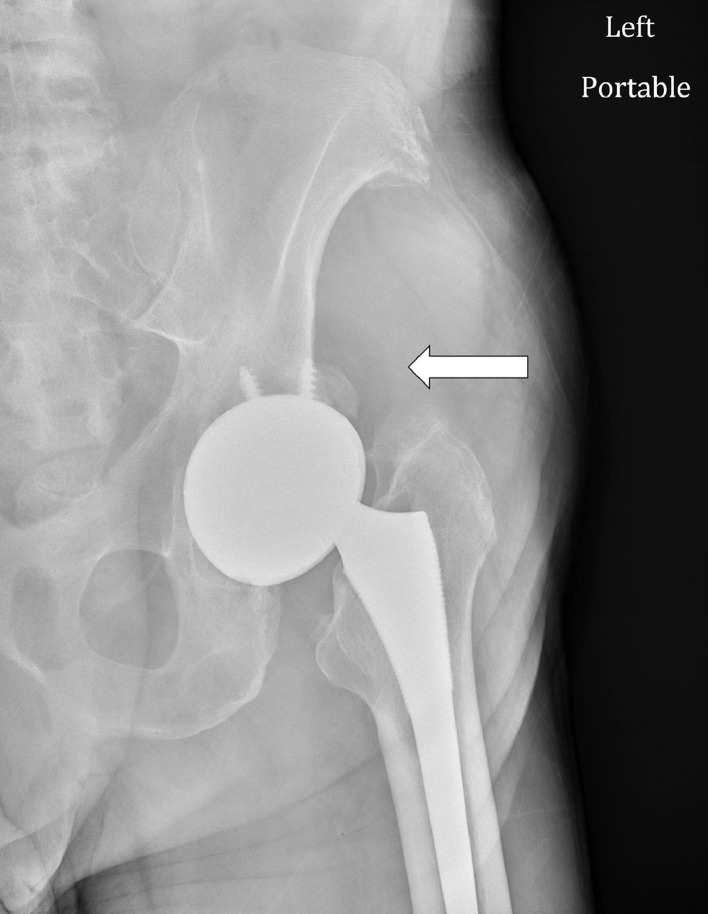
AP radiograph of the left hip shows a subtle semicircular lucency along the superolateral aspect of the hip (arrow) indicative of the “bubble sign.” There is a total hip arthroplasty without acute fracture, hip dislocation, periprosthetic lucency, or soft tissue gas. There is minimal heterotopic ossification along the superolateral left acetabulum.
